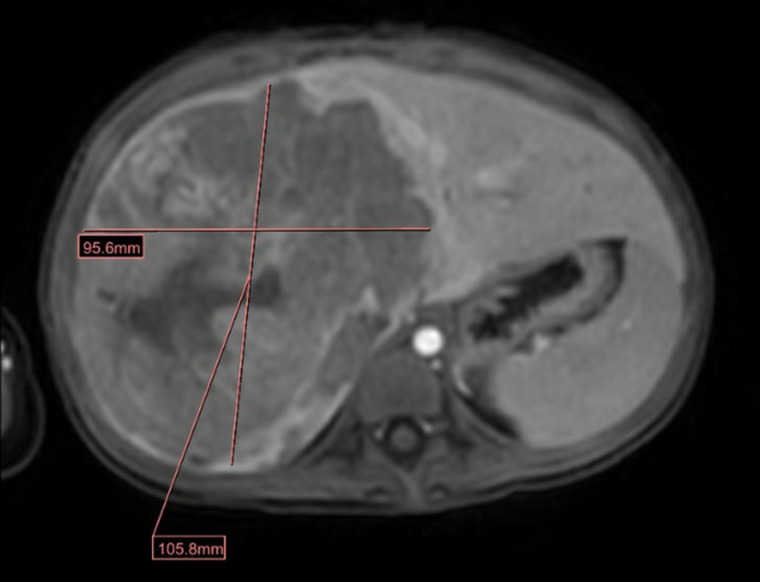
A magnetic resonance image (MRI) of the patient's abdomen showing a very large tumor (12.2 × 10.6 × 9.6 cm) in the right hepatic lobe, primarily involving the posterior segment.
